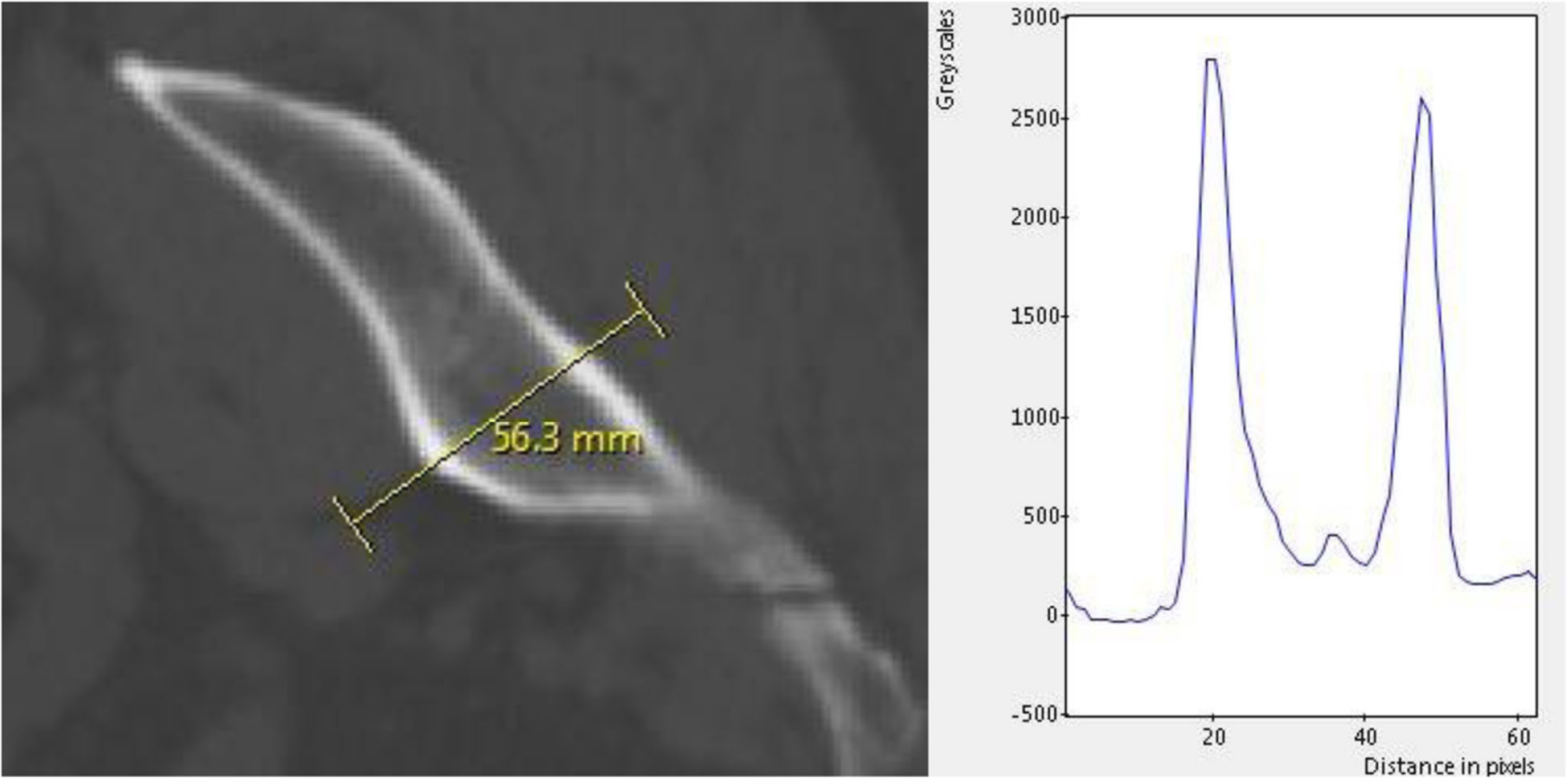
Exemplary grayscale values of the ilium from the CT scan
